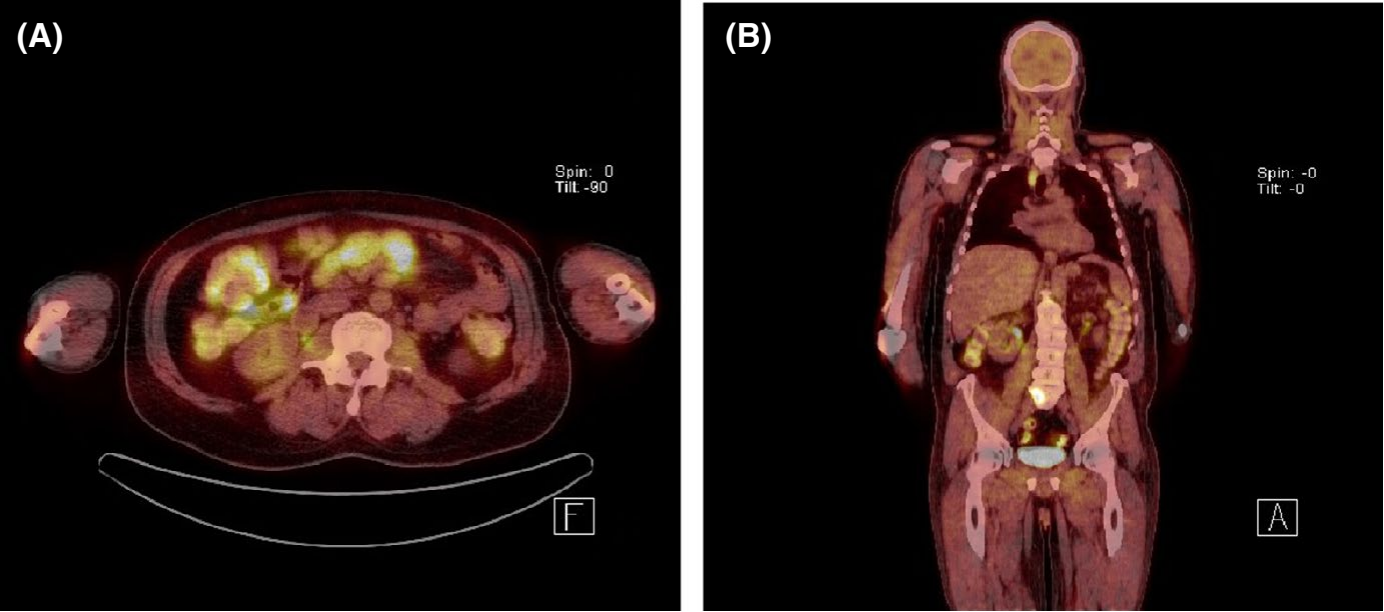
(a) The most active nodal disease is in the central small bowel mesentery (SUVmax 8.7). (b) Nodal involvement below and above the diaphragm and L3 bone involvement
